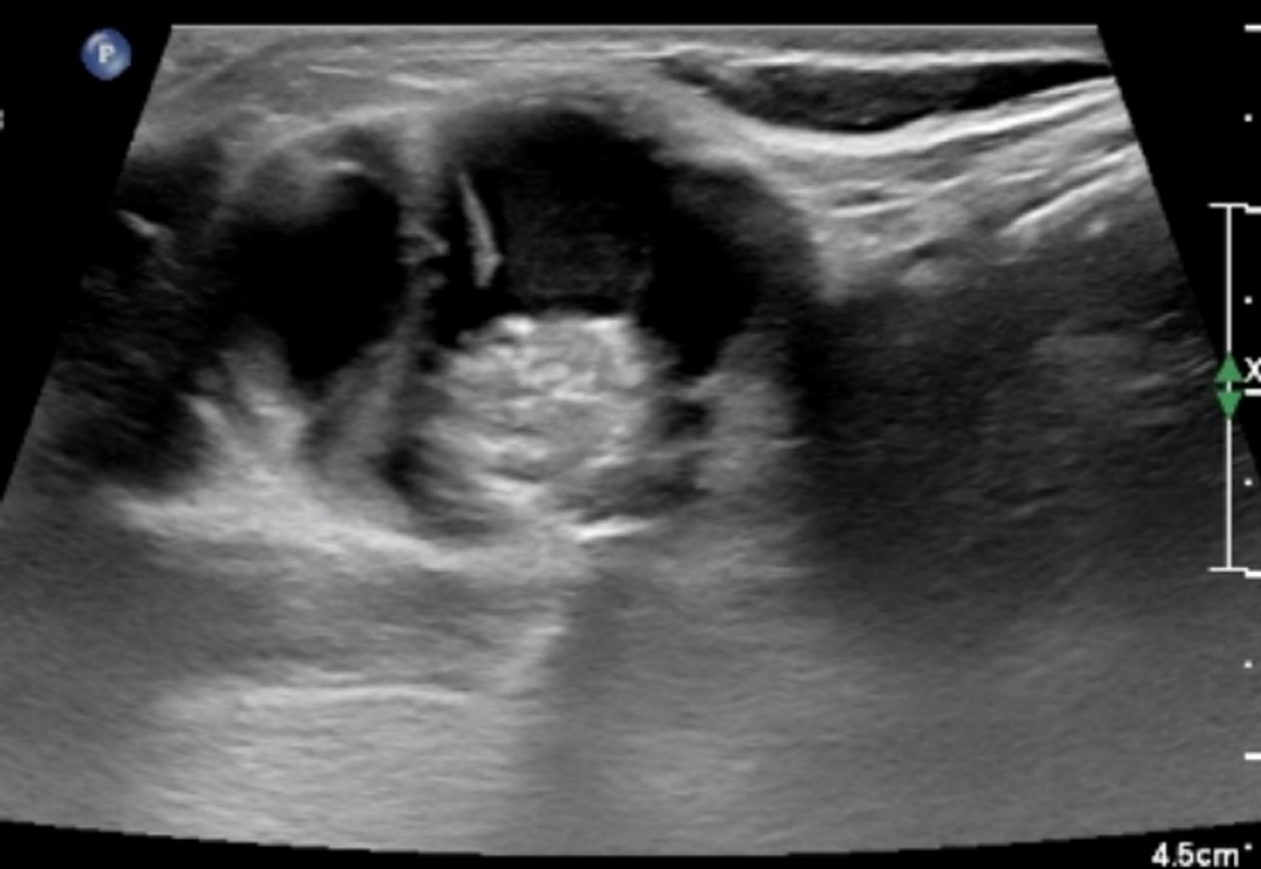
Ultrasound image showing complex solid cystic mass with visible septations.
